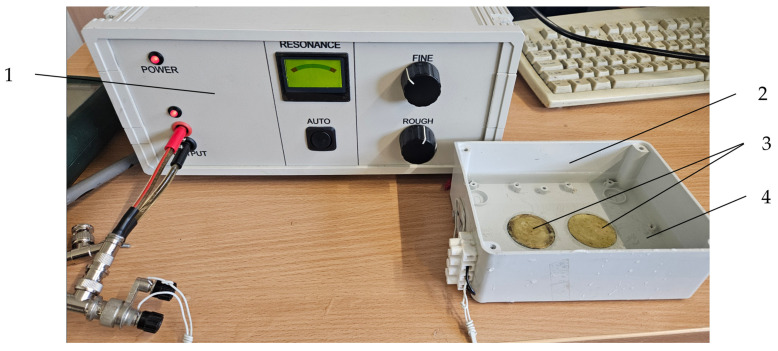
Ultrasonic bath with signal controller: 1—signal controller; 2—ultrasonic bath; 3—piezo elements; 4—distilled water.
